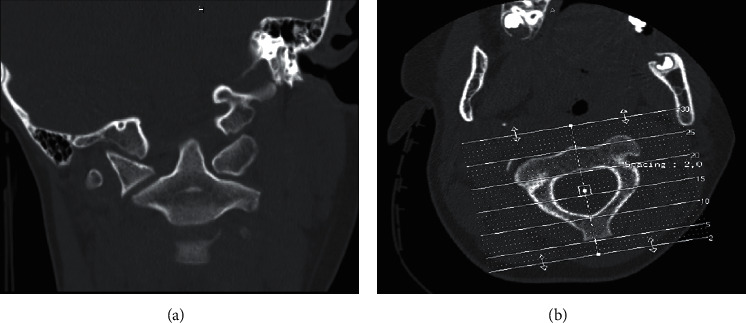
CT scans of our patient 1. (a) The anteroposterior view shows the asymmetry of the lateral masses of the atlas with respect to the axis. (b) The transversal view shows the rotation of the atlas on the axis.
